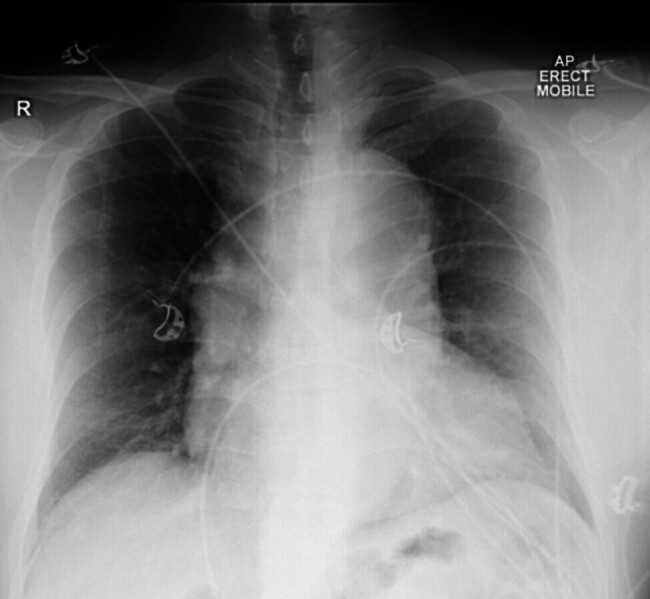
A chest X-Ray - Showing presence of mediastinal widening.
